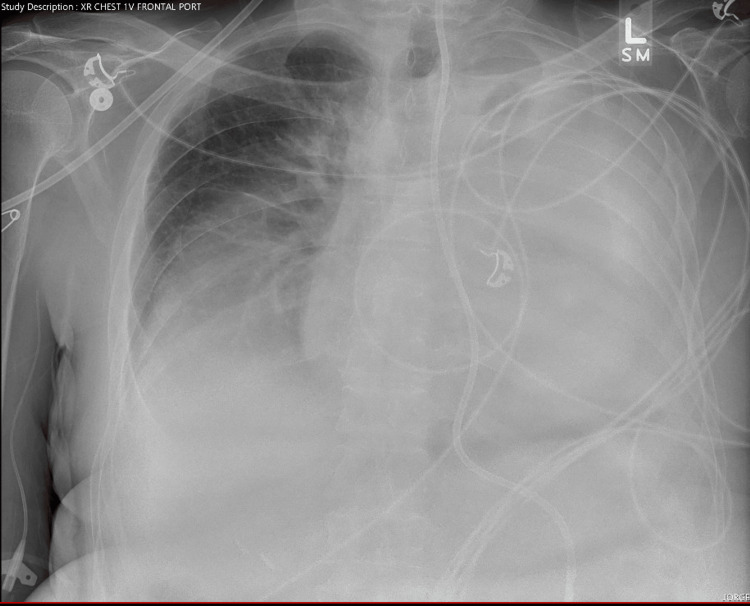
Chest radiograph obtained prior to nasogastric tube insertion.
